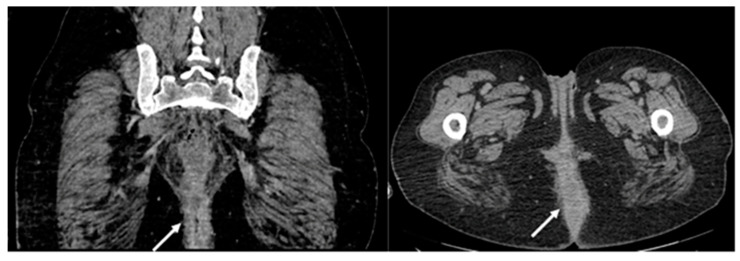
Pelvic CT depicting perineal invasion of the tumor (write arrows).
